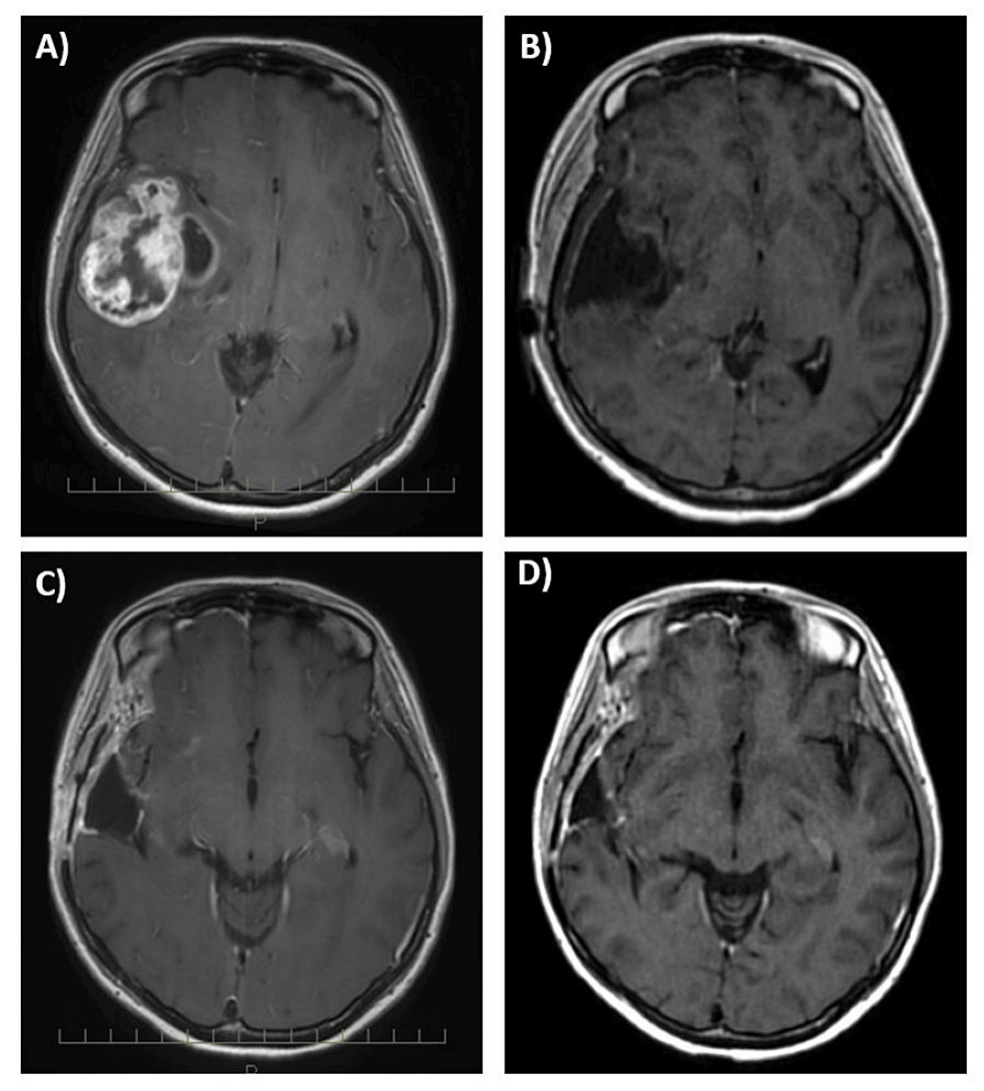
MRI brain findings (Case 1) (A) Preoperative MRI with contrast showing ring-shaped contrast-enhanced lesion was observed in the right temporal lobe; (B) Postoperative MRI with contrast after the tumor was surgically removed via craniotomy, showing total resection was achieved; (C) Two months after the start of maintenance therapy, no contrast lesions noted; (D) Four months after the start of maintenance, no contrast lesions noted.
